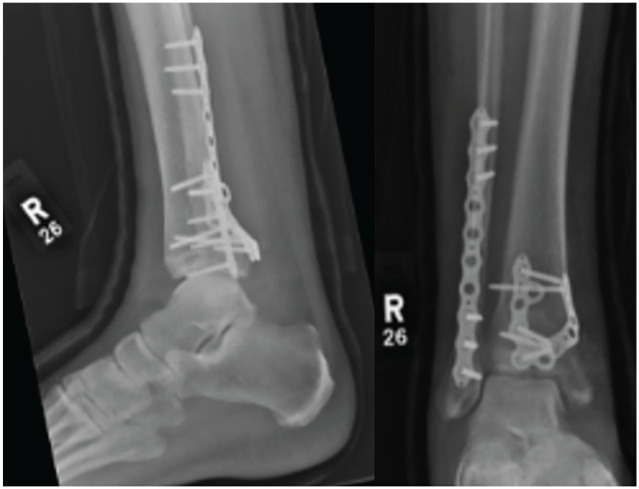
Radiograph showing (Volition) type IIB (posteromedial tibial plate) in situ.
